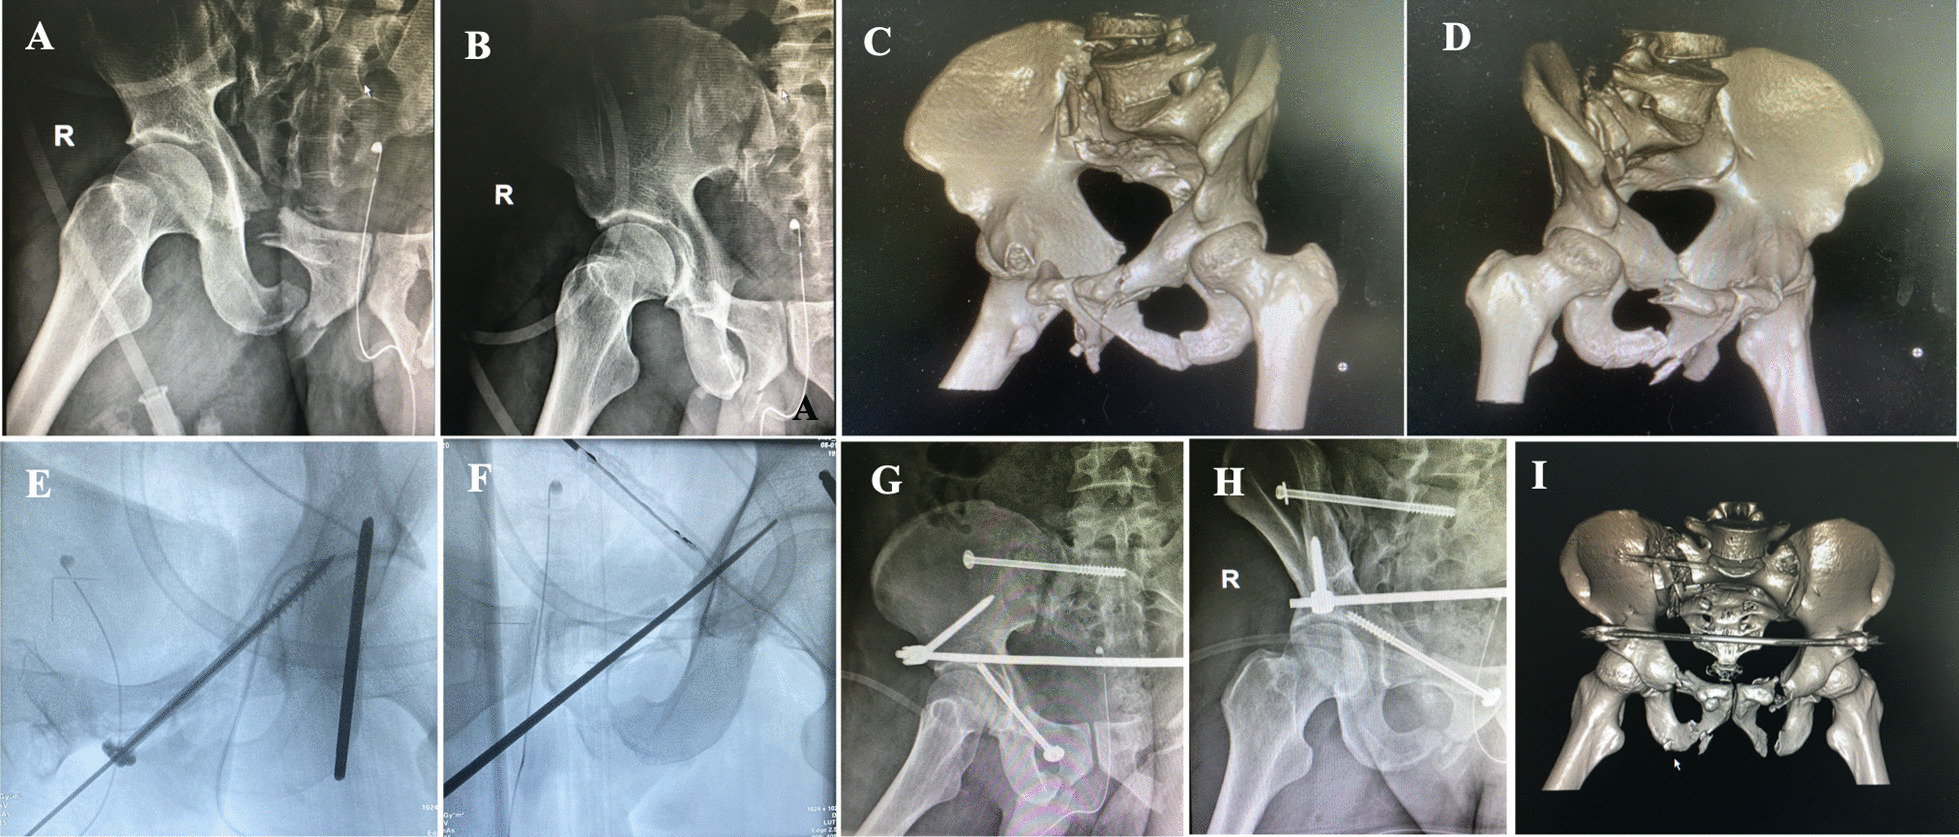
A 58-year-old male patient with pelvic fracture caused by falling from height, the displacement of right pubic ramus fracture was significantly greater than 2 cm, and surgical treatment was performed. Displacement of left pubic ramus fracture was less than 2 cm, and conservative treatment was performed. A–D Preoperative X-ray and CT film indicated displacement of the pubic ramus fracture is greater than 2 cm. E Intraoperative fluoroscopy indicated the pedicle finder entering a good bony channel and fracture obtained a initial reduction. F The fracture obtained a well reduction and compression when cannulated compressive screw was implanted. G–I Postoperative X-ray and CT film indicated the fracture was well reduction
